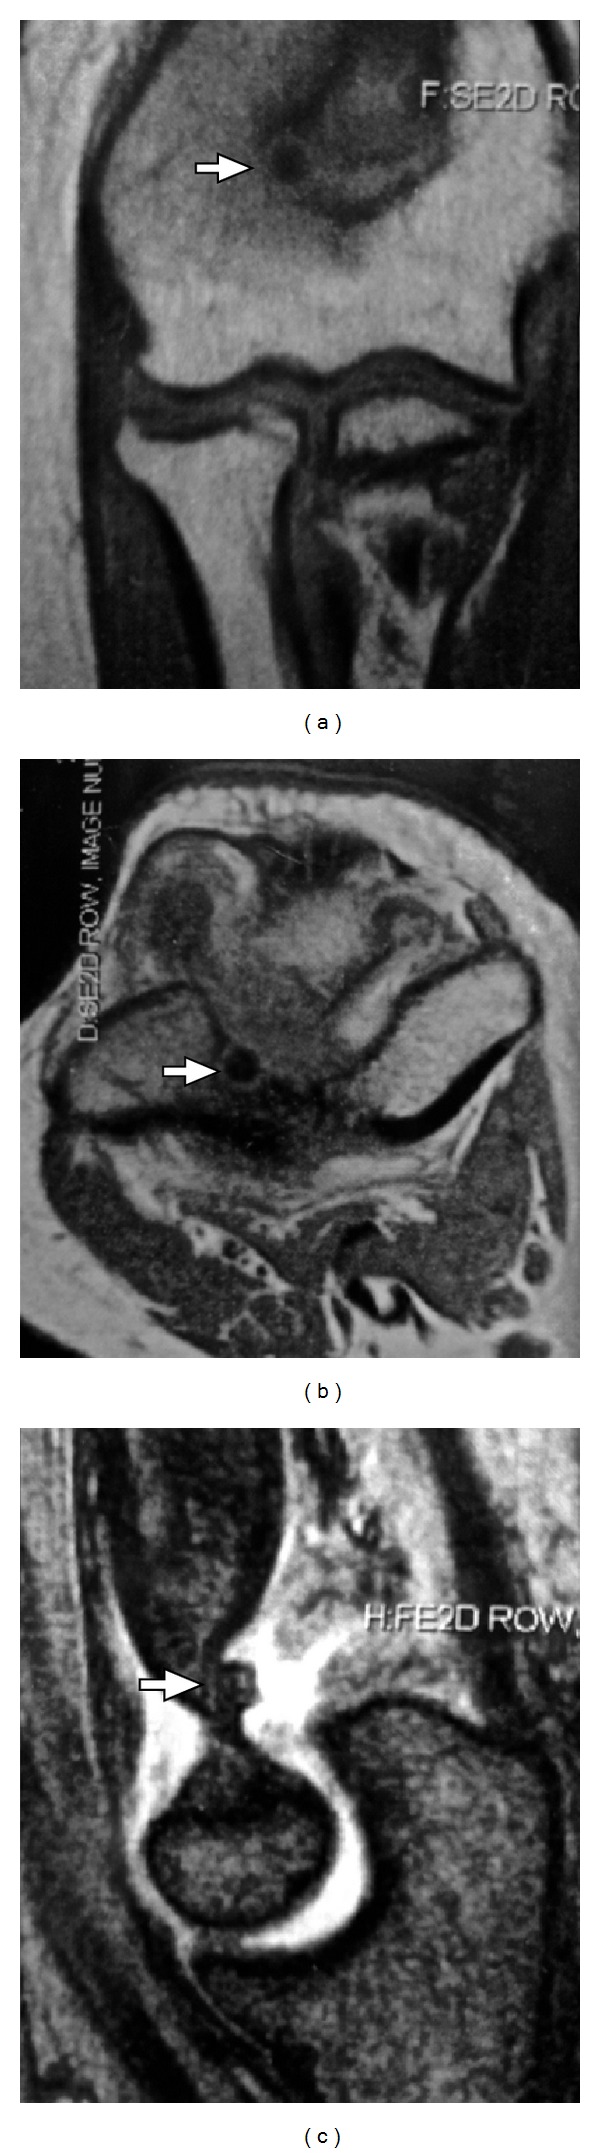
Magnetic resonance image of the nidus located subchondrally and a marked sclerotization of the surrounding bone. No cortical lesion indicating the presence of OO. Signs of mild synovitis were present.
